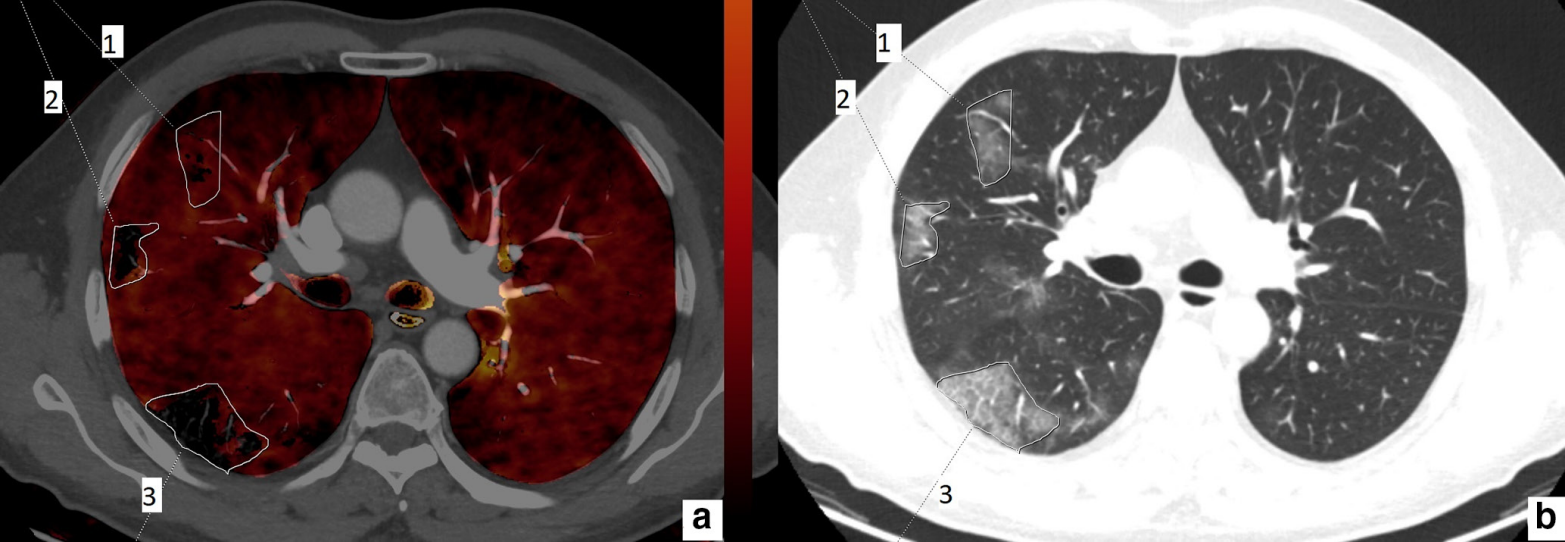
61-year-old male. Axial perfusion blood volume (a) and computed tomography (b) images. In lesion 1, ground glass opacity area is larger than the perfusion deficit. In lesions 2 and 3, the perfusion deficit and ground glass opacity areas are the same.
